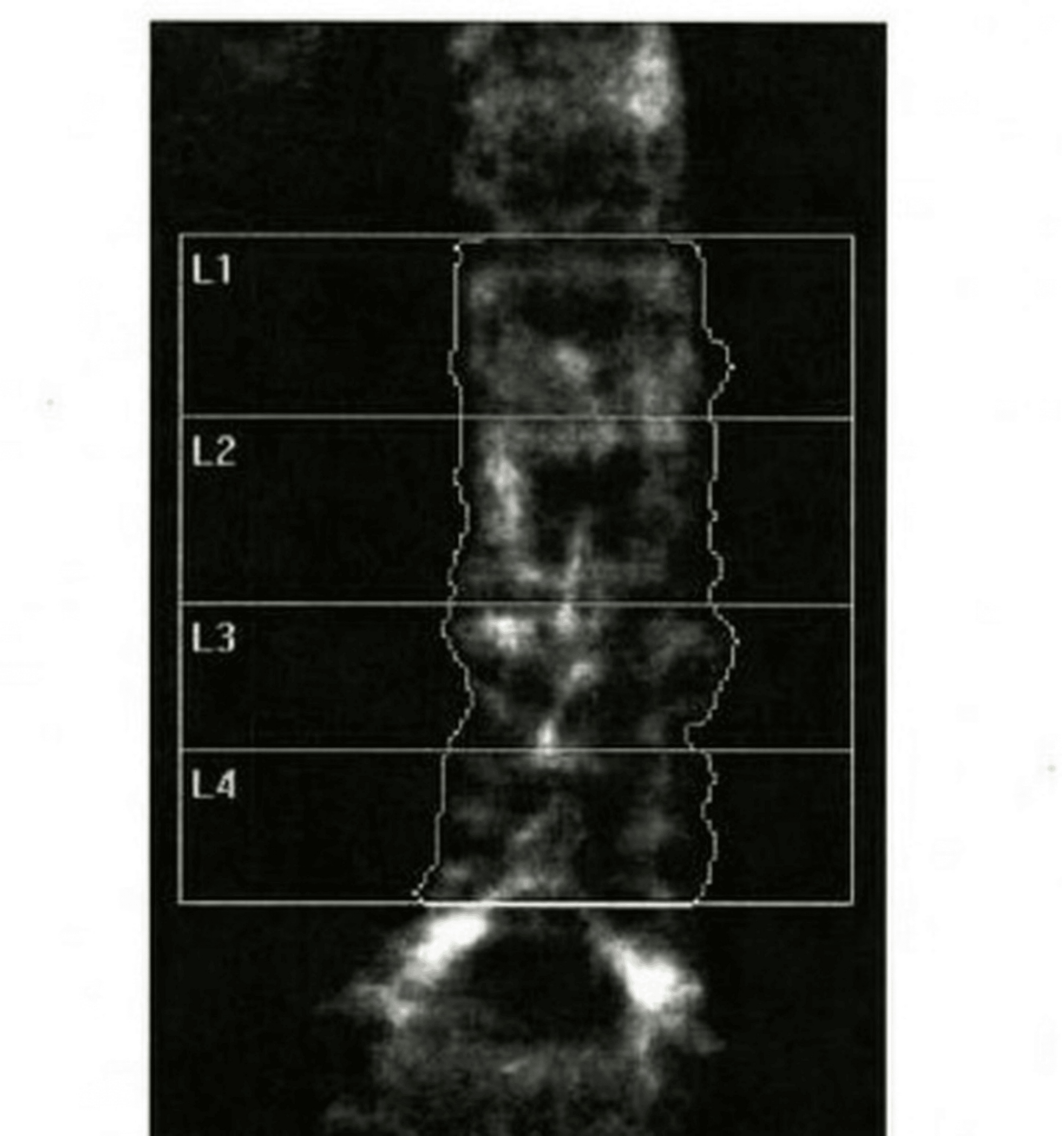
Anterior-posterior lumbar spine DXA scan (L1-L4) The lumbar spine DXA scan (L1-L4) was acquired using a Hologic densitometer (Hologic Inc.). Quantitative DXA measurements are presented separately in Table 4. DXA, dual-energy X-ray absorptiometry
